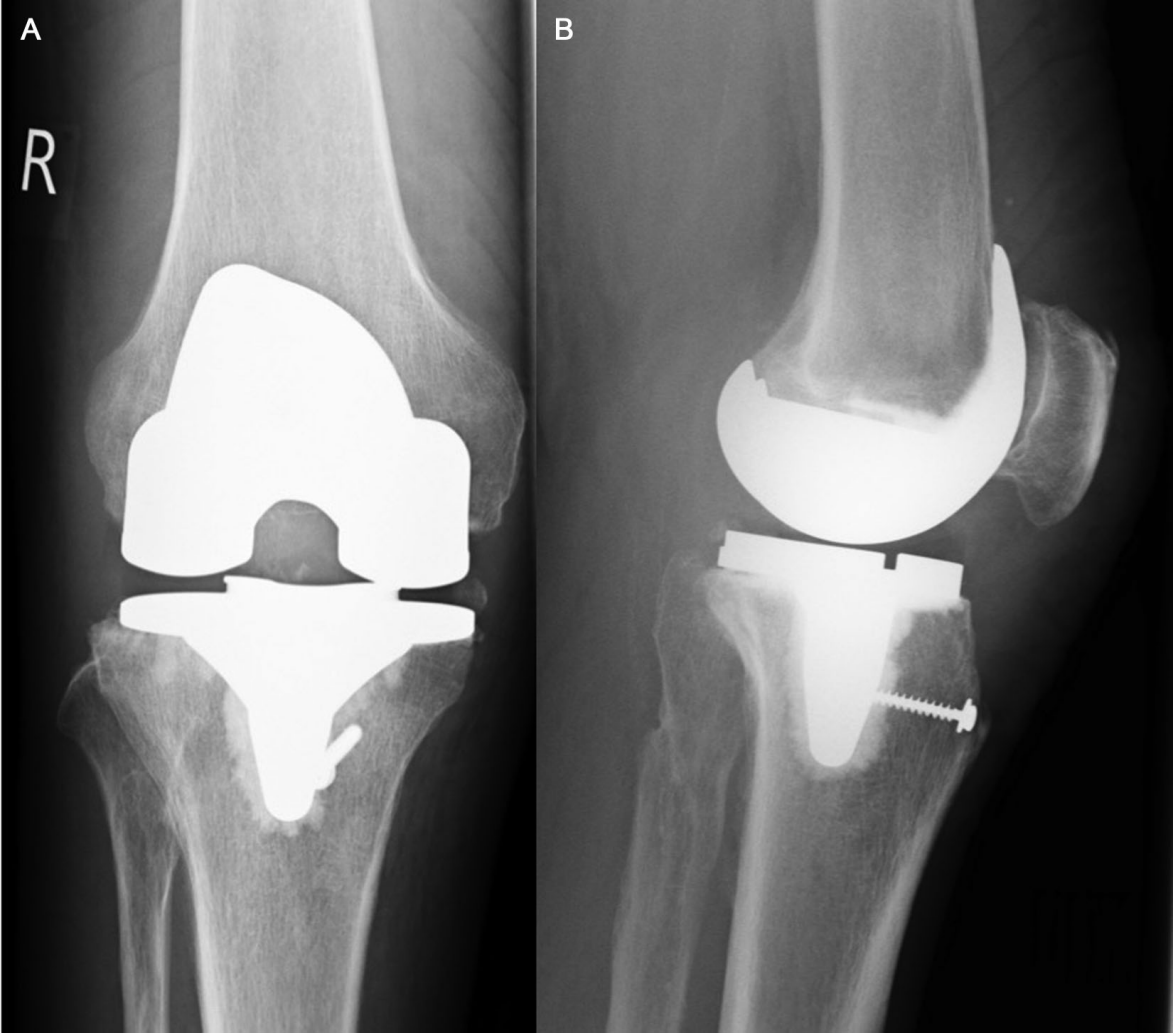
(A,B) Marlex Mesh fixation. Postoperative antero-posterior (A) and lateral (B) x-ray views of the knee three months after Marlex Mesh reconstruction. Regarding its distal fixation, a trough was created in the proximal-anterior aspect of the tibia. Additionally, a lag screw was placed across the mesh (as described under “2.2 Surgical Technique and Rehabilitation Protocol”).
